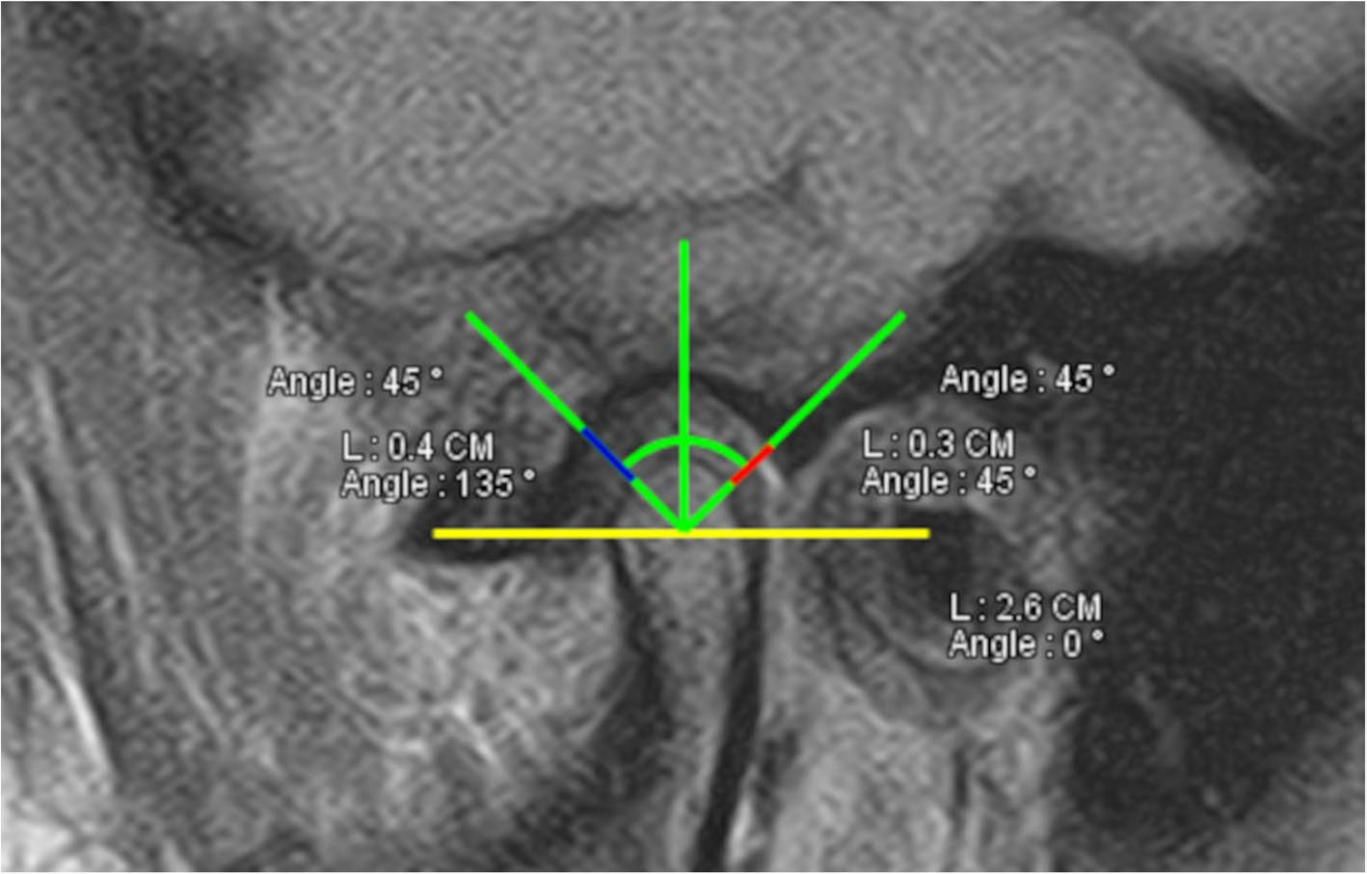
Application of Rammelsberg et al. method on MRI for measuring the condylar position
